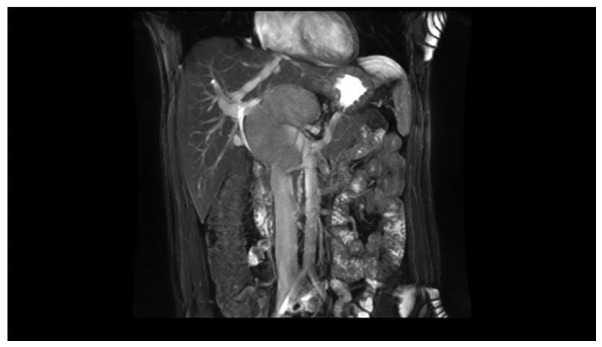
Coronal gadolinium-enhanced T1-weighted fat-suppressed magnetic resonance image shows significant enhancement of the mass at the pancreas head and uncinate process.
